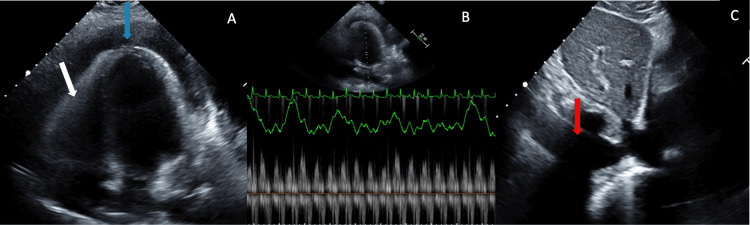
Transthoracic echocardiogram findings. (A) Apical window showing circumferential large pericardial effusion (blue arrow) with evidence of diastolic right ventricular wall collapse (white arrow); (B) excessive respiratory variation in the mitral valve spectral doppler velocities; (C) dilated and nonreactive inferior vena cava (red arrow).
